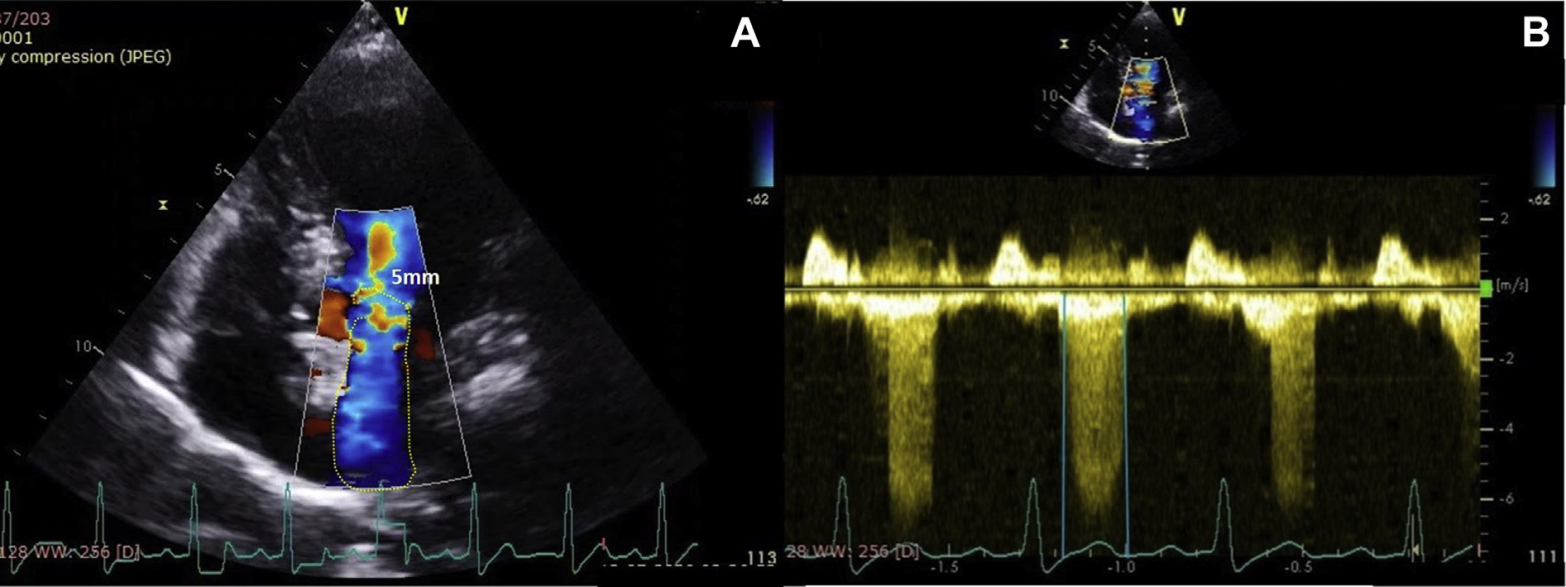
(A) Two-dimensional TTE apical four-chamber view with color Doppler evaluation. The mitral regurgitation in mid-late systole was demonstrated to be moderate grade with a regurgitant jet area of 50% and a vena contracta of 5.0 mm (B) Two-dimensional TTE with continuous-wave Doppler across the mitral valve showing a mid-late systolic mitral regurgitation (*teallines*) and a peak velocity of 6.1 mm Hg.
